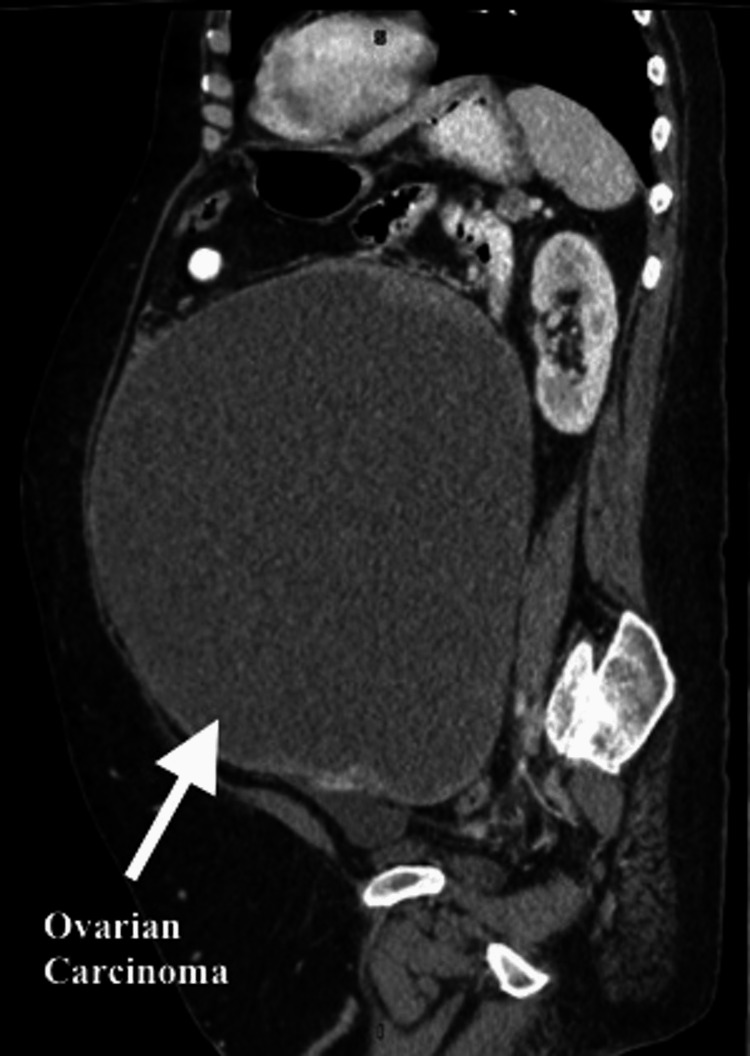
Sagittal view of the patient’s abdominal CT scan illuminating the severity of tumor size (arrow). CT: computed tomography
